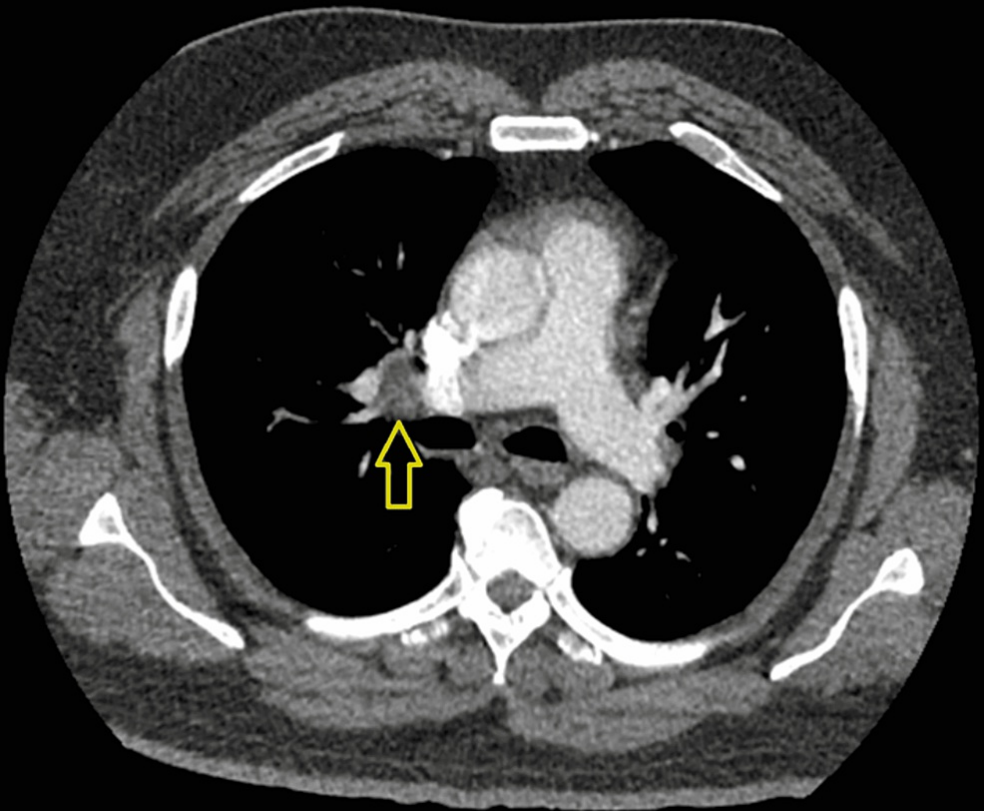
CT imaging showing a right lower lobe segmental pulmonary artery embolism.
